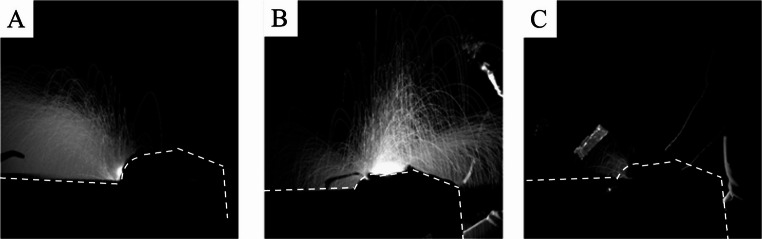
LED-illuminated photographs of aerosol and droplet dispersion without surgical intraoral suction or extraoral vacuum, (A) air turbine handpiece, (B) ultrasonic bone-cutting device, (C) implant motor
